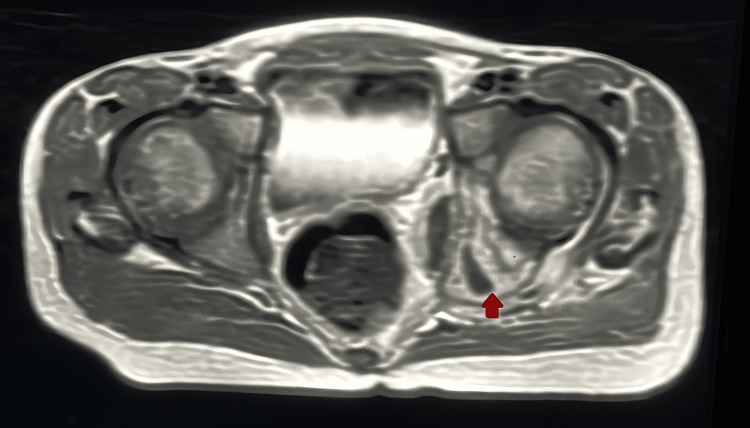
MRI showing sizable collection at the hip joint
